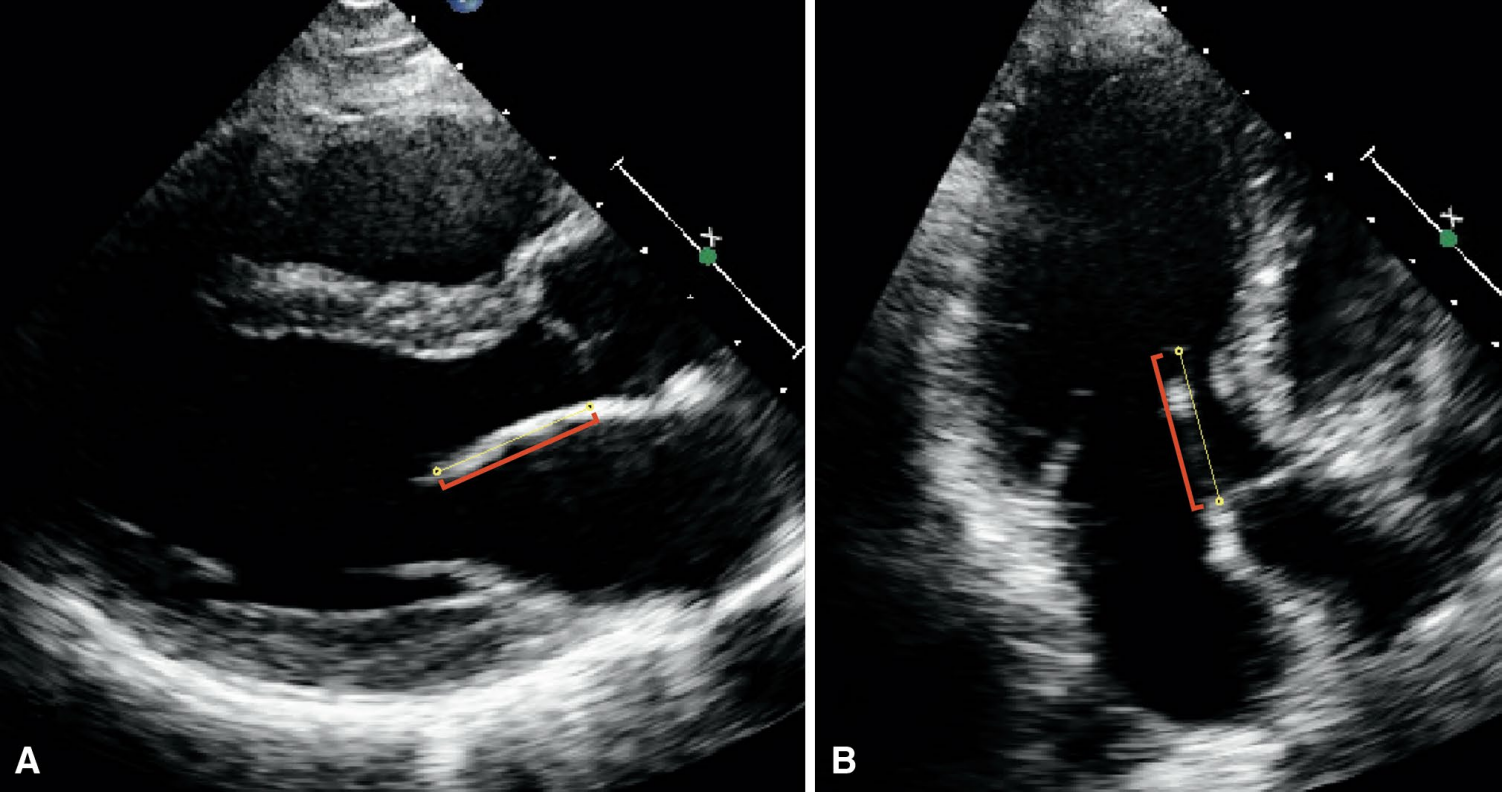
Example of anterior mitral valve leaflet length (AMVL) measurements in a hypertrophic cardiomyopathy gene mutation carrier without hypertrophic changes. In the parasternal long-axis view, a the AMVL measured 26 mm, and in the apical three chamber view, b the AMVL measured 26 mm
